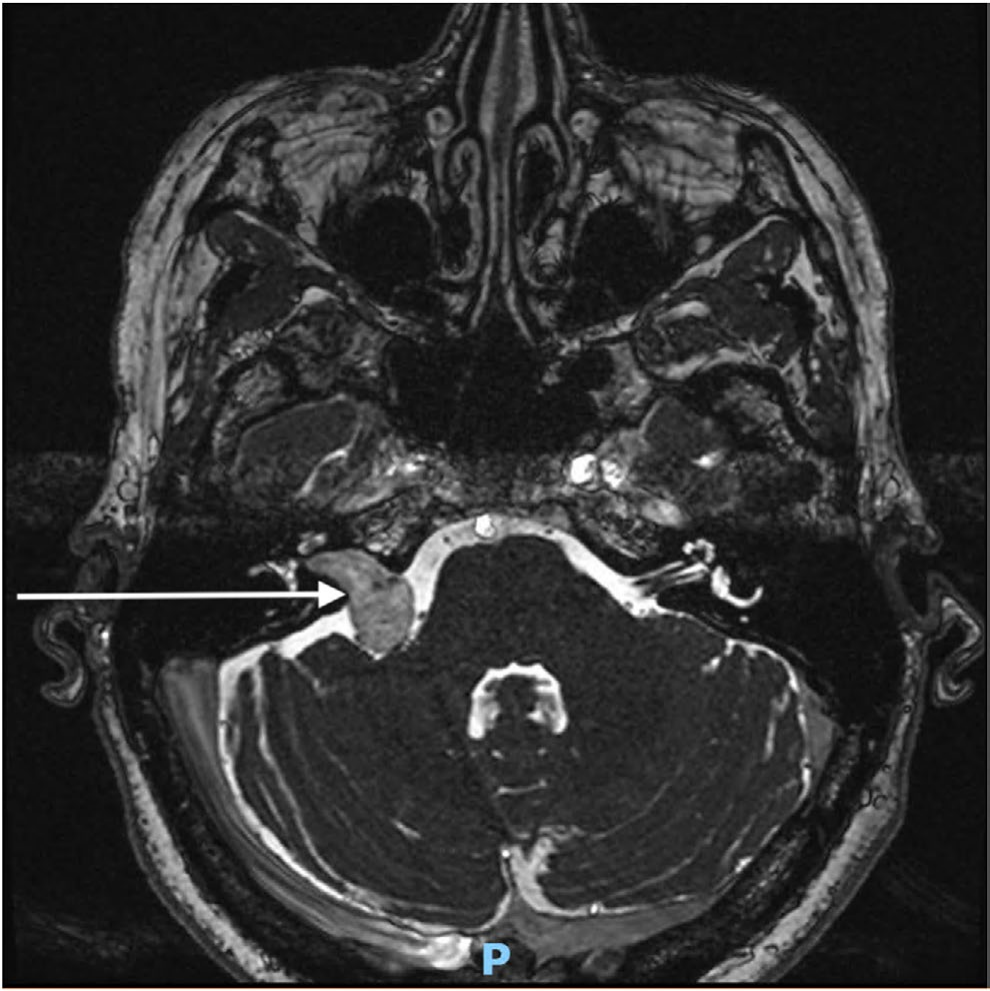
Preoperative MRI (T2‐weighted sequence) showing the vestibular schwannoma (white arrow) in the cerebellopontine angle. This tumor was classified as Koos III due to its close contact with the brainstem but without compression of it.
